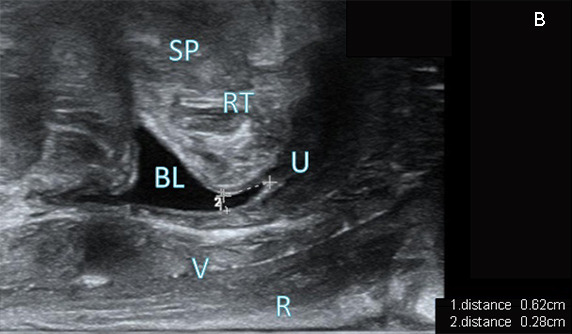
Midsagittal plane obtained by biplanar transrectal ultrasound at the Valsalva state: internal urethral orifice opening in individuals with SUI.
